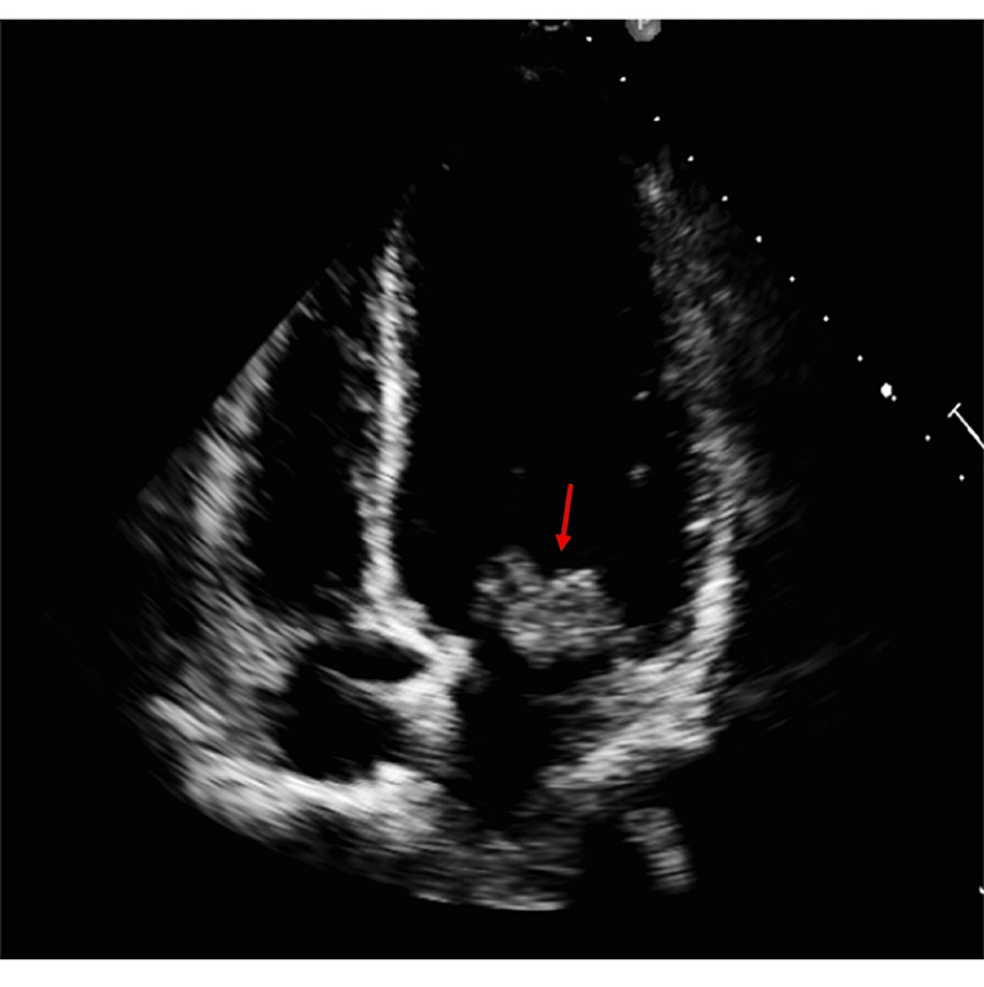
Parasternal long axis showing mitral valve vegetation (red arrow)
